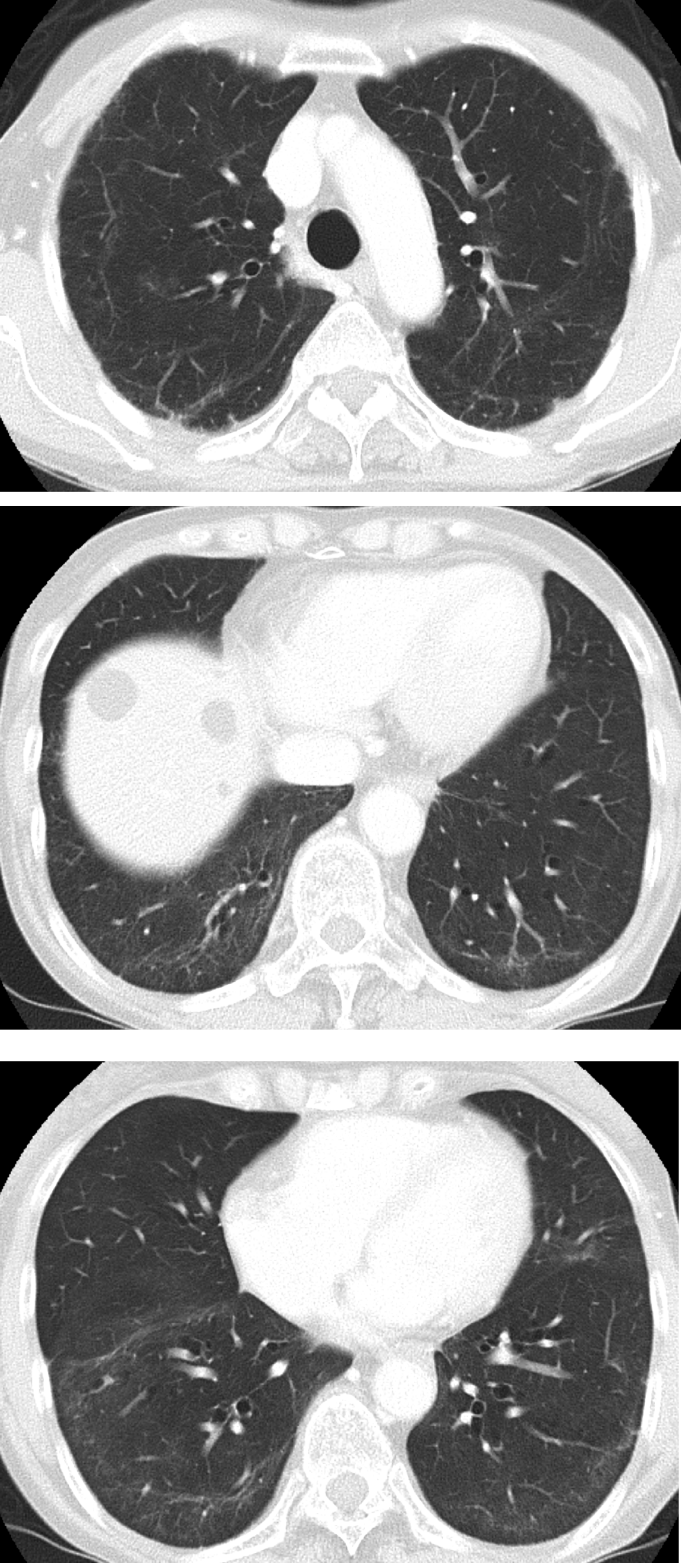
Computed tomography after 14 months from the onset.
